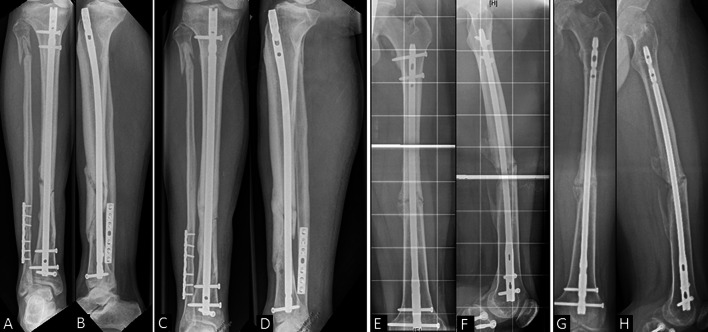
A–D Serial radiographs of a 50-year-old patient after a II° open tibia fracture showing non-union 8 months after primary surgery (A, B) and osseous union 6 months after nail dynamization and fESWT (C, D). E–G A 27-year-old patient suffered from a closed femur fracture with consecutive non-union 1.5 years later (E, F). Treatment of the clinical control with nail dynamization was not successful after 6 months (G, H)
